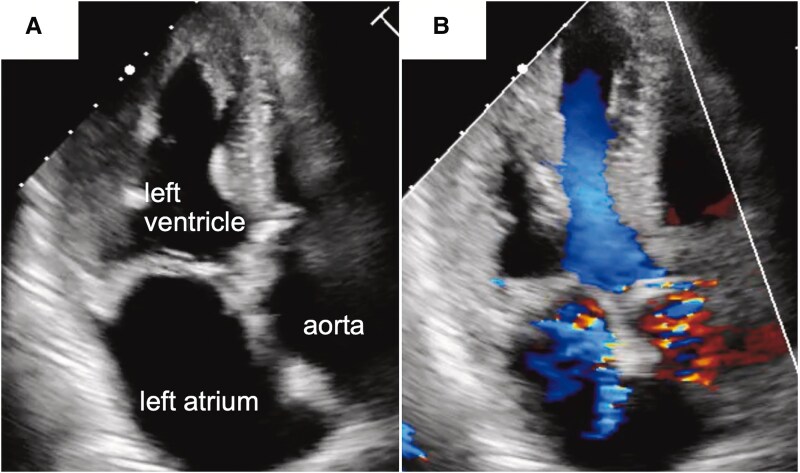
Echocardiographic views. (*A*) Pre-transcatheter aortic valve implantation transthoracic echocardiography showing severe aortic stenosis and left ventricular hypertrophy. (*B*) Doppler imaging at the same level demonstrating moderate mitral regurgitation.
